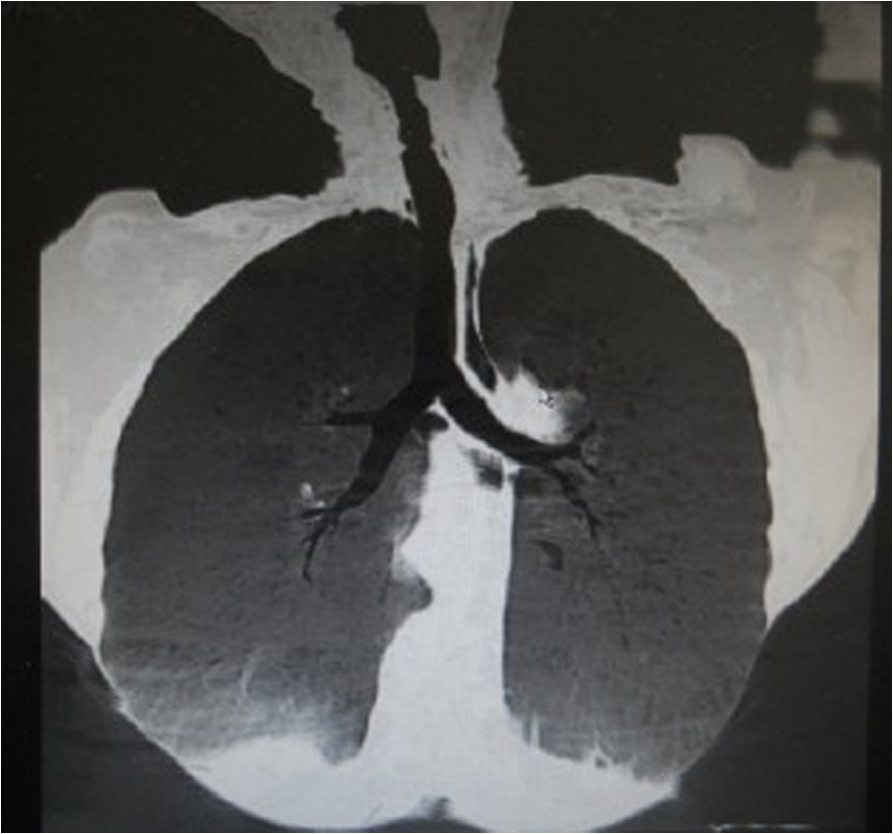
CT scan of the neck at 2 months after surgery. The tracheal injury has healed well with no proliferation of granulation tissue.
